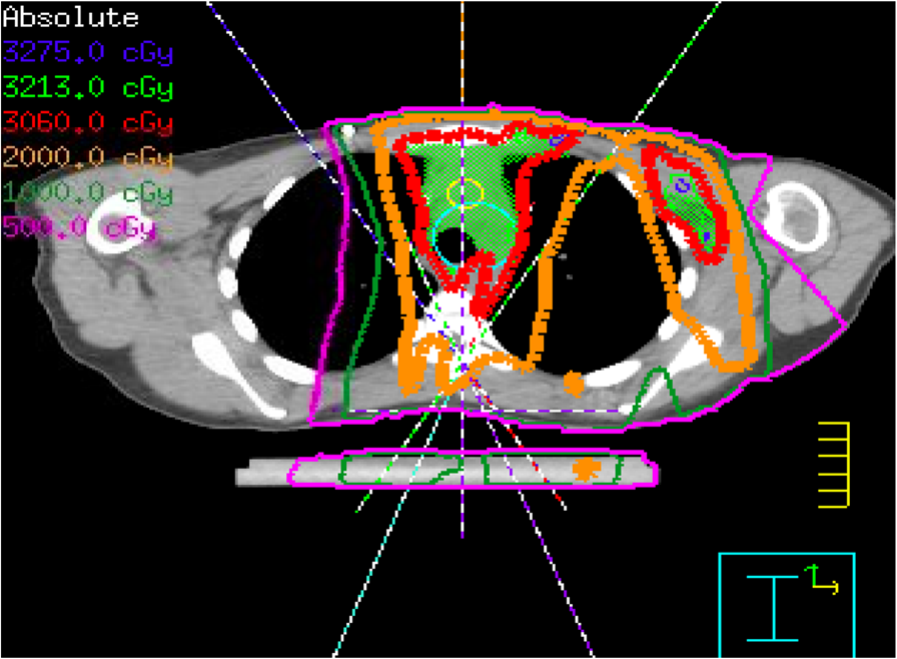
Axial CT treatment-planning scan shows beam angles for the butterfly technique.
